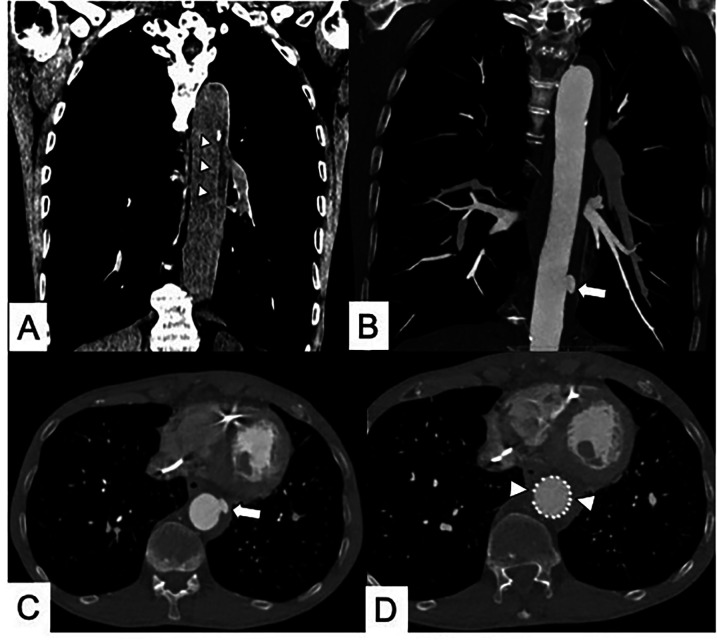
(A) Coronal multiplanar reformation CT in subacute Type B IMH (arrowheads). (B,C) One-month CT follow-up shows the appearance of an ulcer-like projection (ULP) treated (D) with thoracic endovascular aortic repair (TEVAR) (arrowheads).
